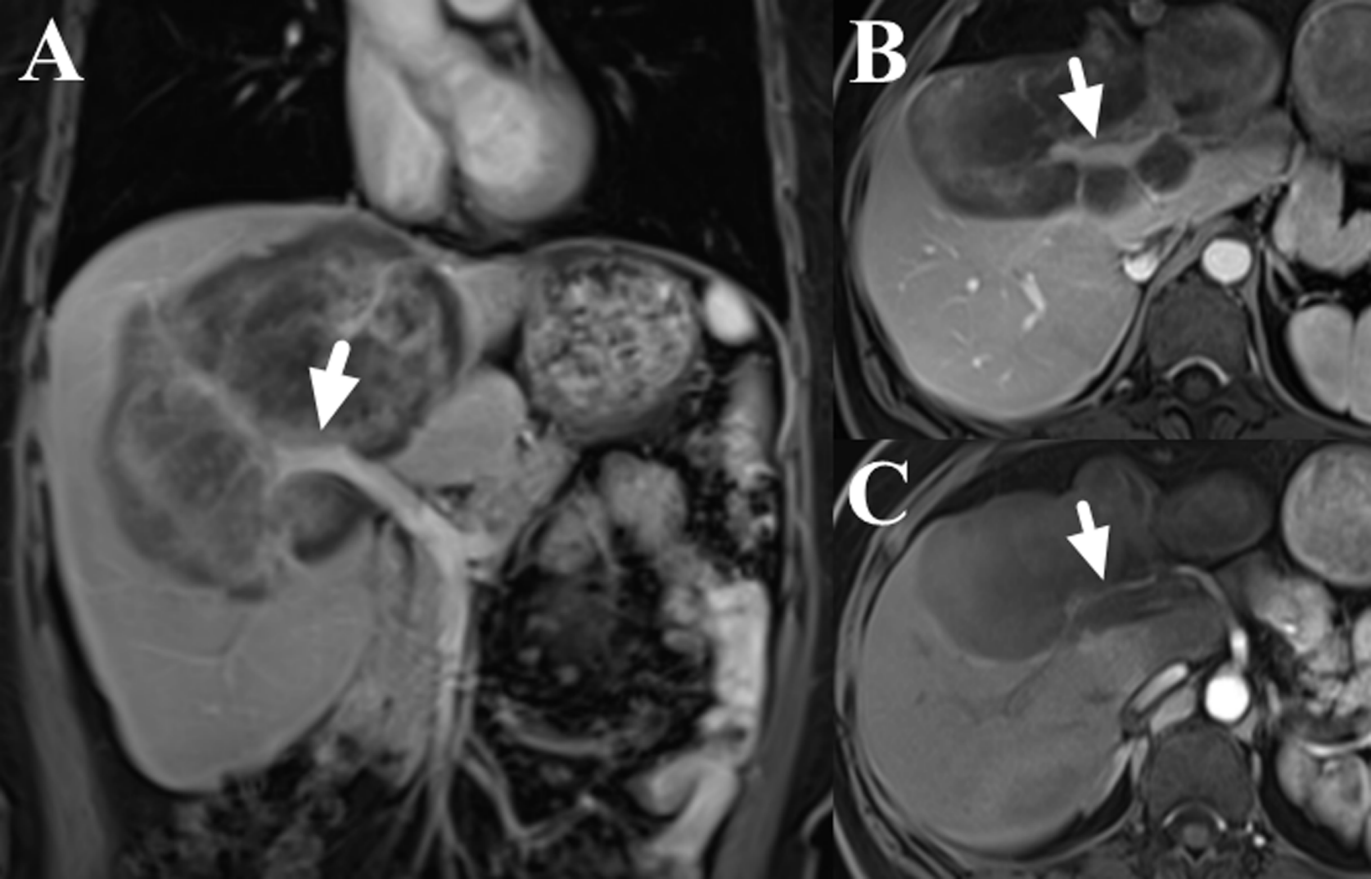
Contrast-enhance images of liver MRI (dose: 0.1 mmol/kg; injection rate: 2.0 ml/s; TR/TE: 3.6/1.7 ms; slice thickness: 5 mm; image matrix: 256 × 192; field of view: 40 × 44 cm; the scanning delay times were 20s, 60s and 180s for the arterial, portal and delay phases, respectively), which showed the involvement of right branch of portal vein (A, B, marked with arrows) and right hepatic artery (C, marked with arrow).
